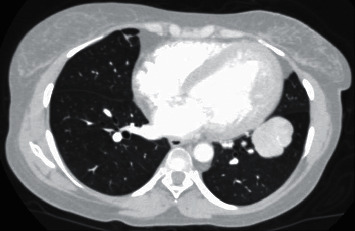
Contrast-enhanced computed tomography (CT) of the chest showing a lung mass measuring 4.4 × 4.0 × 4.0 cm.
